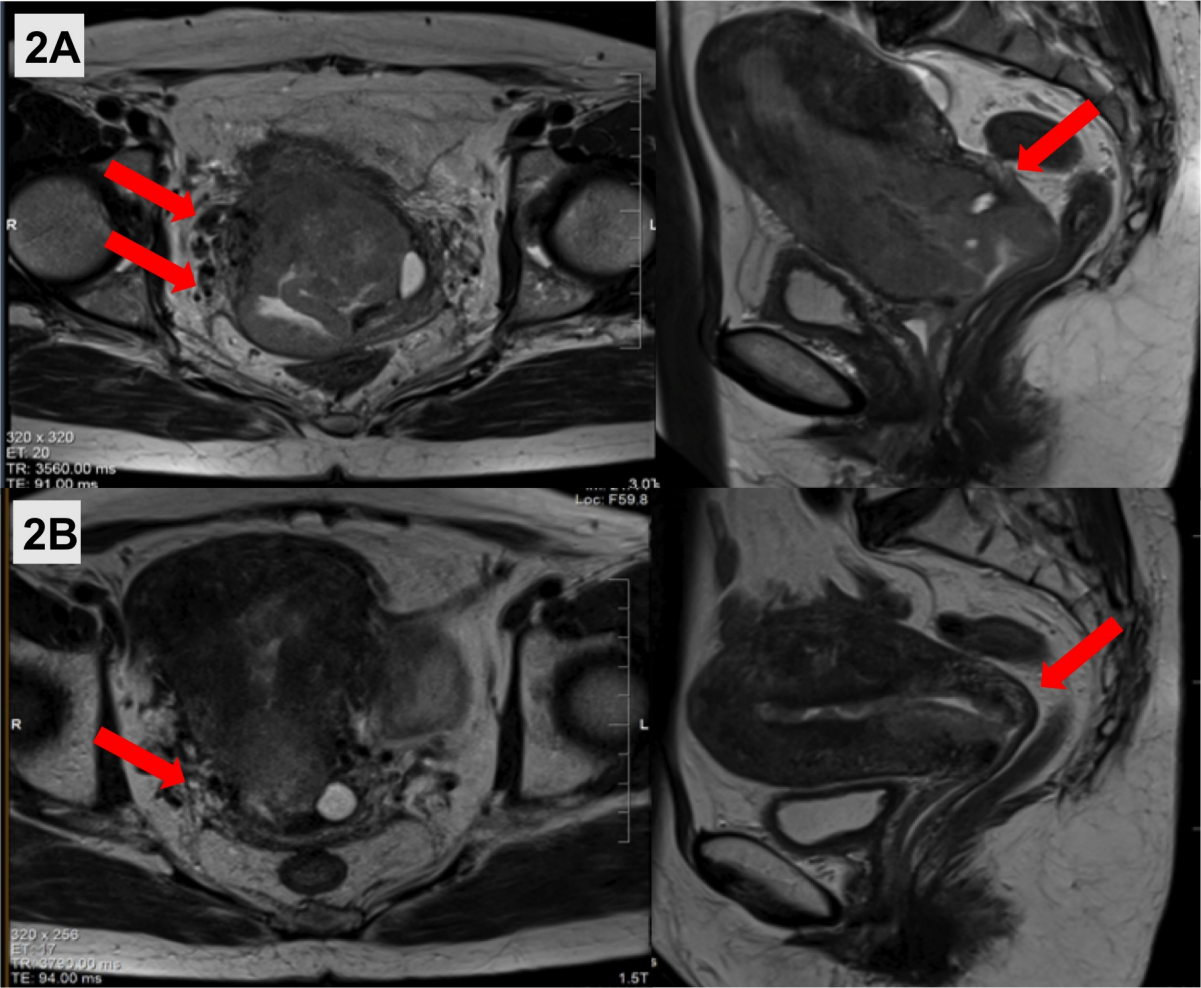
(A) Pre-treatment T2-weighted axial and sagittal MRI showed a large uterine mass extending down the cervix and involving the right parametria (red arrows). (B) MRI at the end of external beam radiotherapy showed a good response with minimal residual parametrial involvement (red arrows), rendering the tumor resectable.
